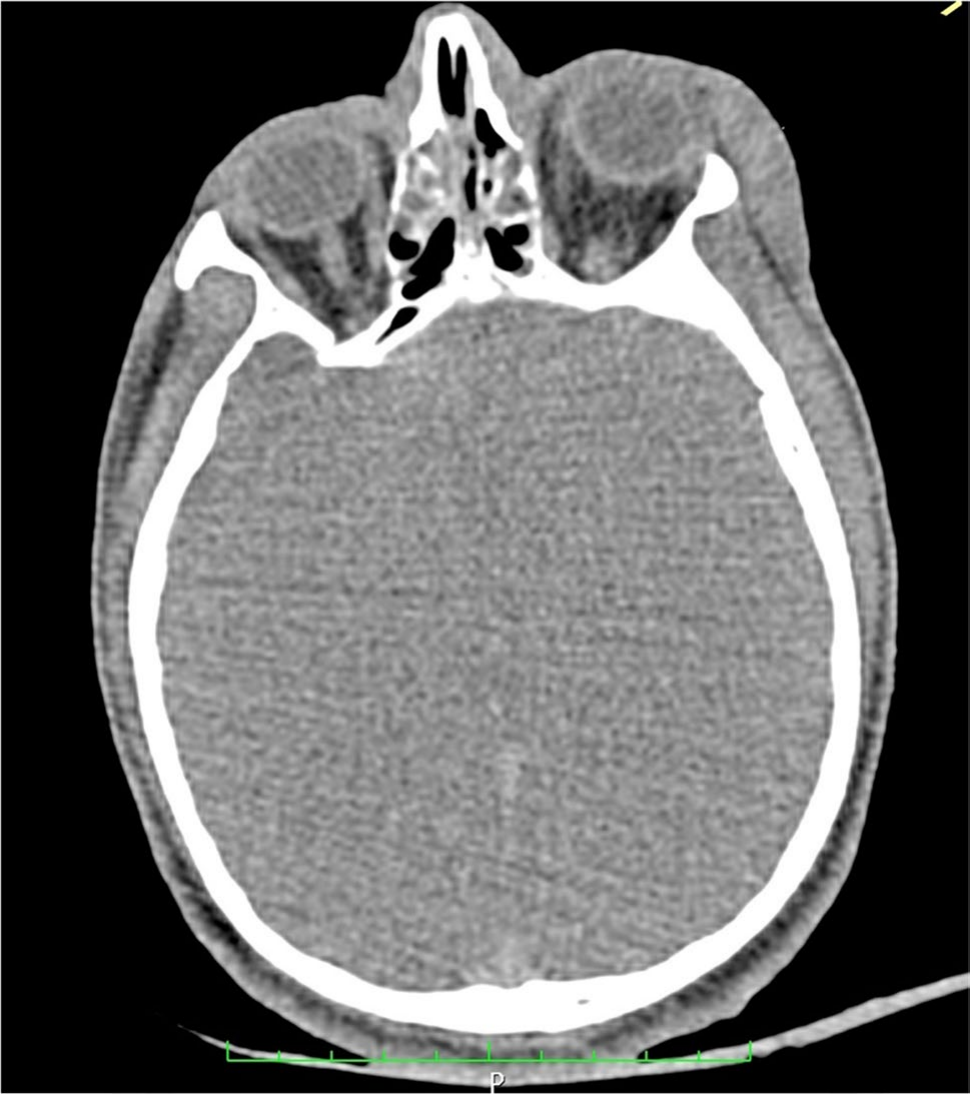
CT image showing massive cerebral edema
